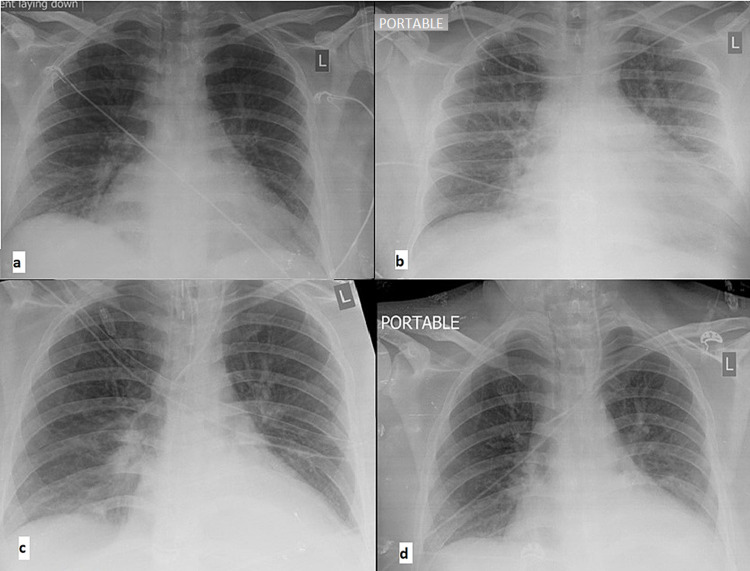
Chest x-rays (a) Admission day, (b) day two, (c) day five, and (d) day eight.
